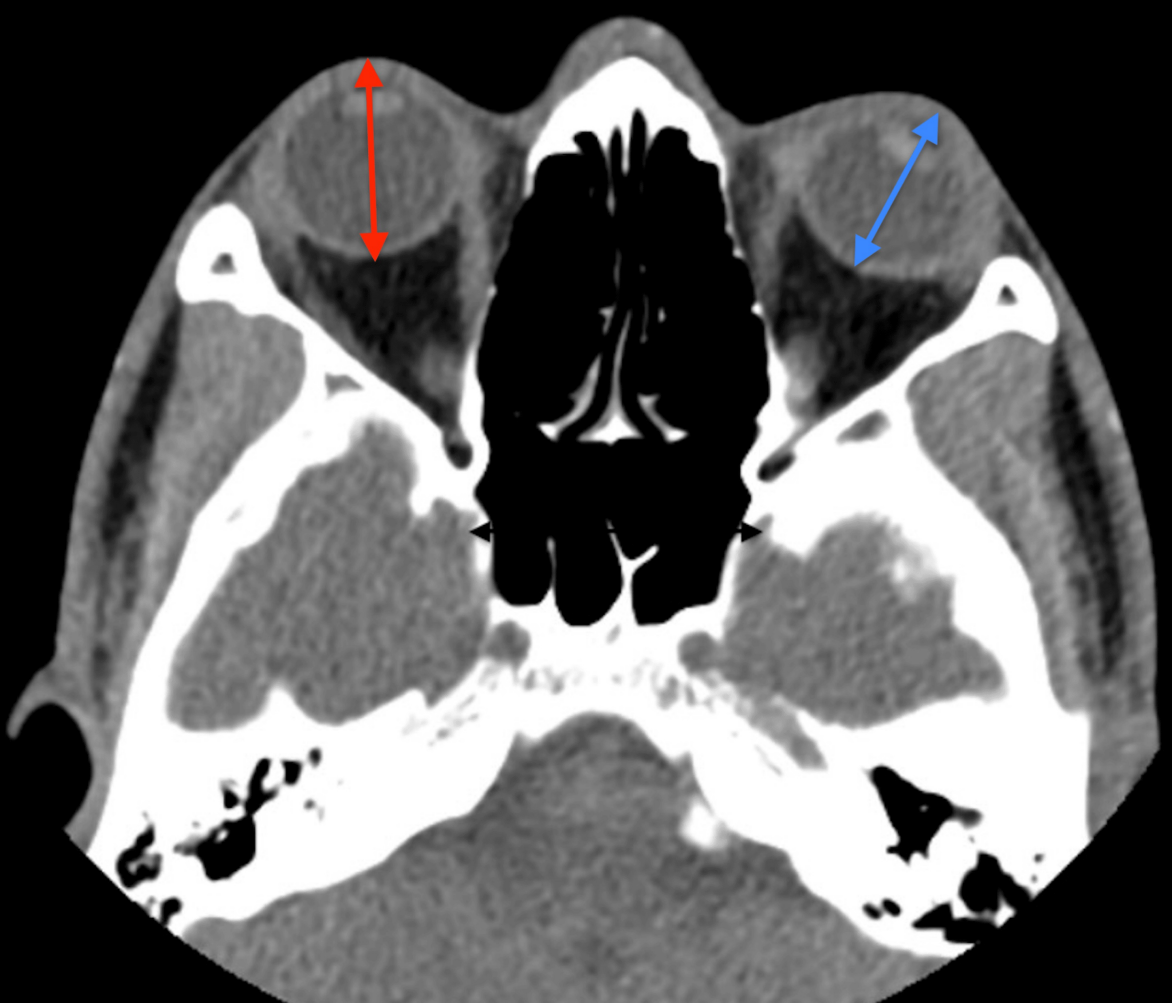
CT orbit without contrast revealing normal anteroposterior diameter of the right orbit (red arrow) and shortening of the anteroposterior diameter of the left orbit (blue arrow), confirming globe rupture
